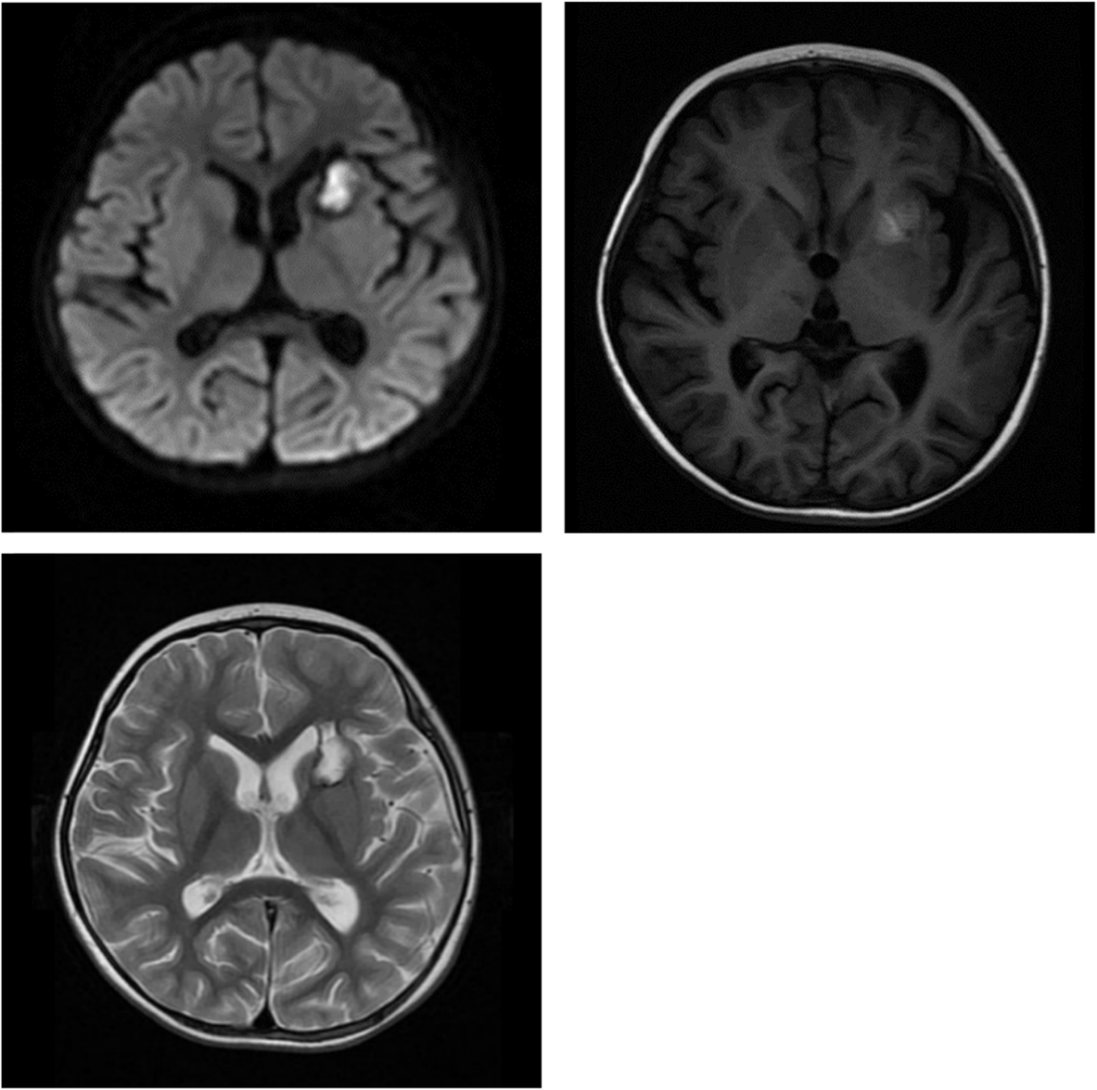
Hemorrhagic stroke at left basal ganglia seen on cerebral magnetic resonance image (MRI)
